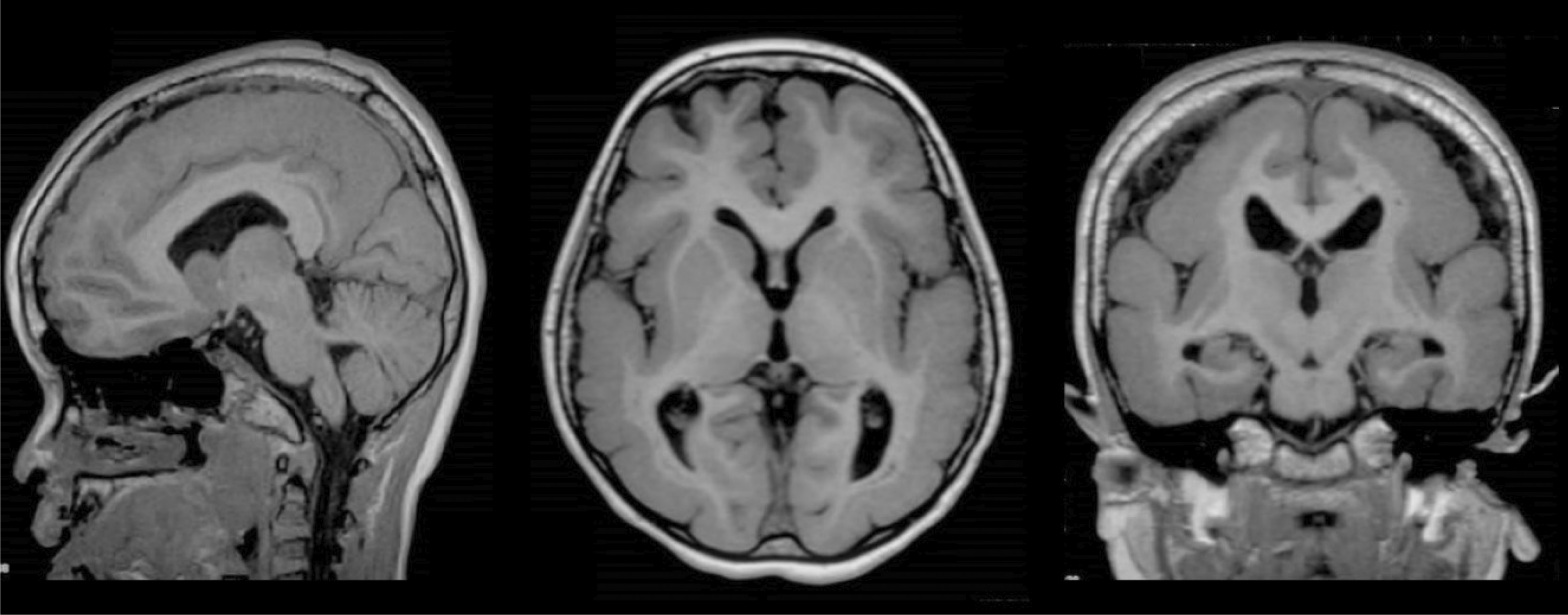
MRI at 8 years of age. Sagittal, axial, and coronal T1 images showing extensive pachygyria particularly in the parietal and occipital lobes. The corpus callosum, basal ganglia, brainstem, and cerebellum are normal.
